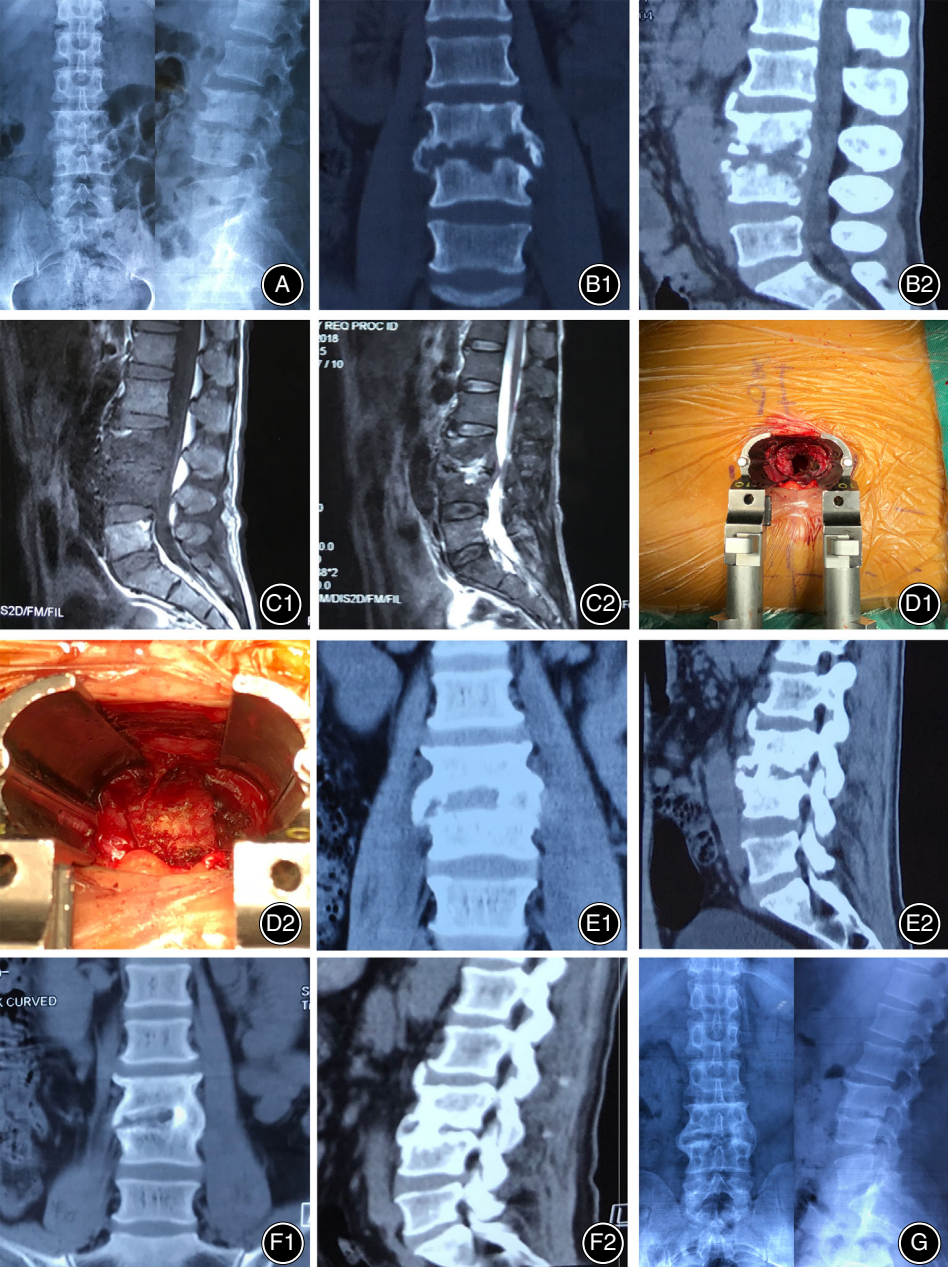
Forty‐eight‐year‐old man, whose complaint was low back and right lower limb pain of more than 2 months. Minimally invasive surgery oblique lumbar interbody fusion (Mis‐OLIF) was performed. Bone defect and paravertebral abscess were seen in the preoperative images and during surgery. (A) preoperative plain radiographs of lumbar; (B1 and B2) preoperative CT images; (C1 and C2) preoperative MRI; (D1 and D2) intraoperative pictures; (E1 and E2) CT images before discharge; (F1 and F2) CT images at 6 months follow‐up; (G) plain radiographs at 6 months follow‐up.
